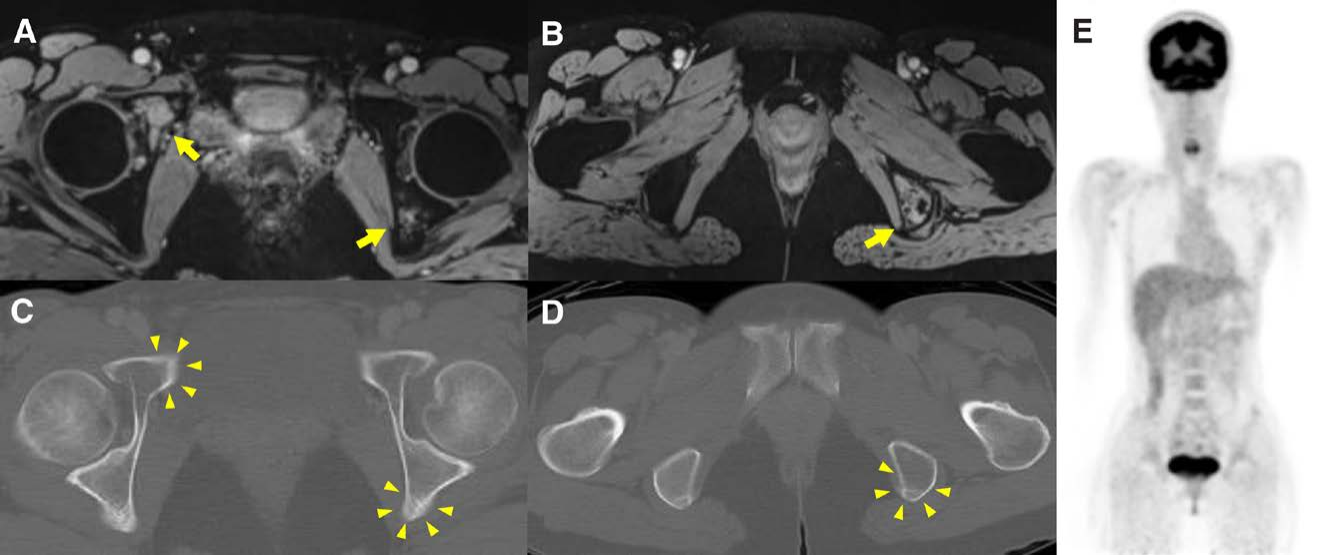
A 39-year-old woman was diagnosed with angiosarcoma of the breast and underwent mastectomy. One year after the surgery, she noticed worsening right coxalgia. On axial Gd-enhanced magnetic resonance imaging (MRI), multiple bone lesions in bilateral acetabulum (A) and the left ischium (B) (yellow arrows) strongly suggested bone metastases. However, axial fluorine-18 fluorodeoxyglucose positron emission tomography/computed tomography (^18^F-FDG PET/CT) showed neither osteolytic destruction nor abnormal uptake around the bilateral acetabulum (C) and the left ischium (D) (yellow arrowheads). Maximum intensity projection also suggested no findings of bone metastases (E).
